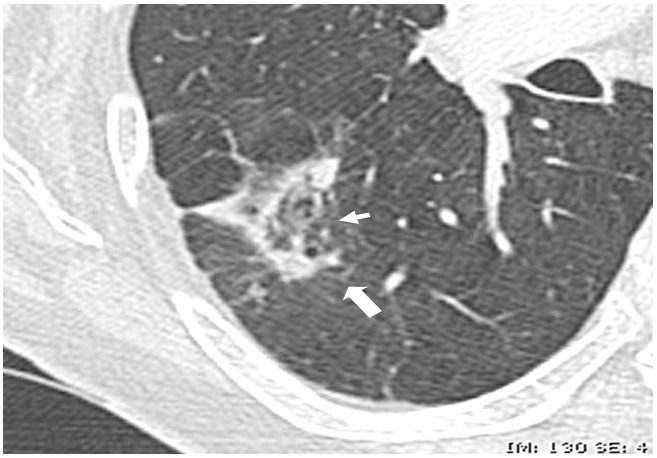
Second post-contrast CT scan, with axial reformations, performed 15 days later showing changes with new central ground glass attenuation (arrow)CT scan examination also showed pulmonary emboli: some clots were central and marginated within the right main pulmonary artery (not shown)
